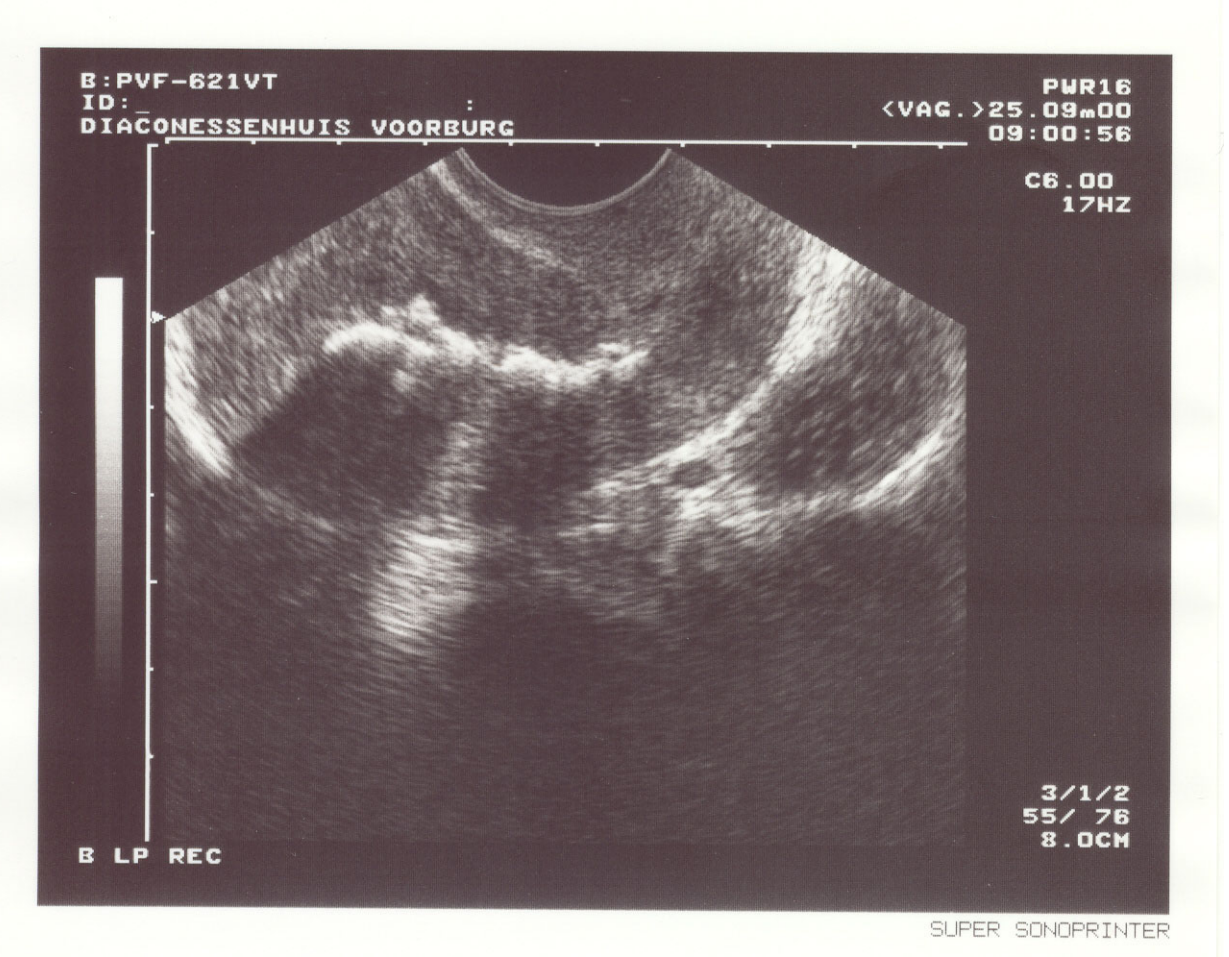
Scarred endometrium revealed on transvaginal ultrasound.
